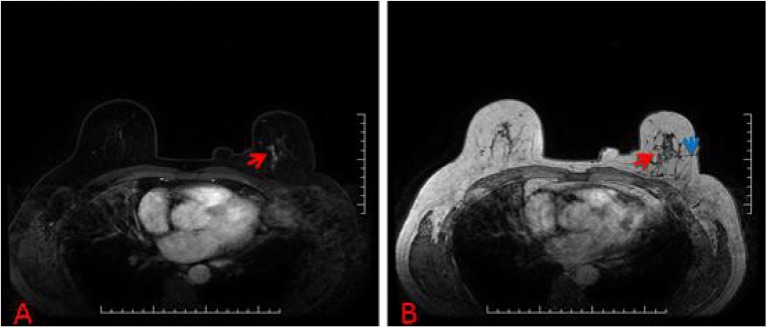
(A) 57-year-old woman, T1-weighted fat suppressed contrast enhanced MRI showed a suspicious lesion at the left breast, and the BI-RADS category was considered as level 4C. (B) The lesion (red arrow) that was revealed to be invasive ductal carcinoma after MRI-guided wire localization (blue arrow) with subsequent surgical biopsy (we chose T1-weighted MRI in order to show the lesion and wire location more clearly).
